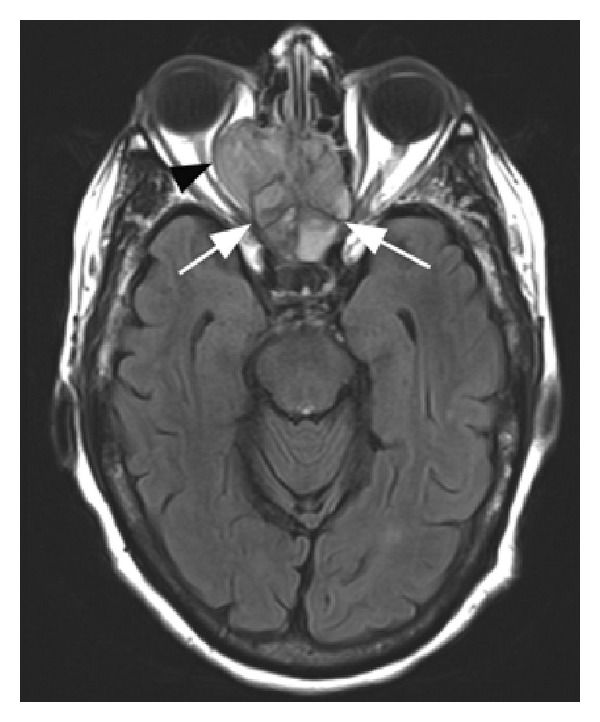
Axial FLAIR T2-weighted brain MRI showing a sinonasal mass involving the ethmoid/sphenoid sinuses (white arrows) with extension into the right orbit (black arrowhead).
